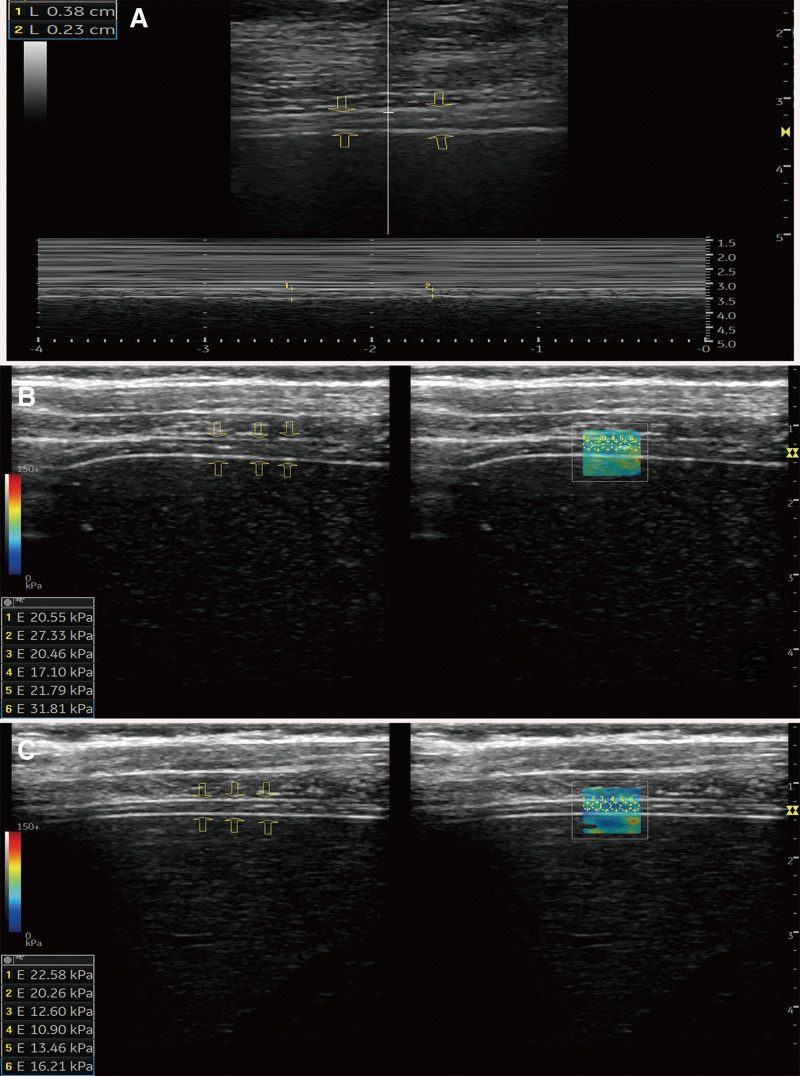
Ultrasound images of diaphragm in a 58-year-old man with AECOPD. (A) The end-inspiratory and end-expiratory diaphragmatic thickness was 0.38cm and 0.23mm, respectively, and the calculated diaphragm thickening fraction was 0.65. (B) The average diaphragm shear wave elasticity at total lung capacity was 23.17KPa in the sampling frame. (C) The average diaphragm shear wave elasticity at functional residual capacity was 16.00 KPa in the sampling frame. After calculation, diaphragm stiffening rate was 0.45. AECOPD = acute exacerbation of chronic obstructive pulmonary disease.
